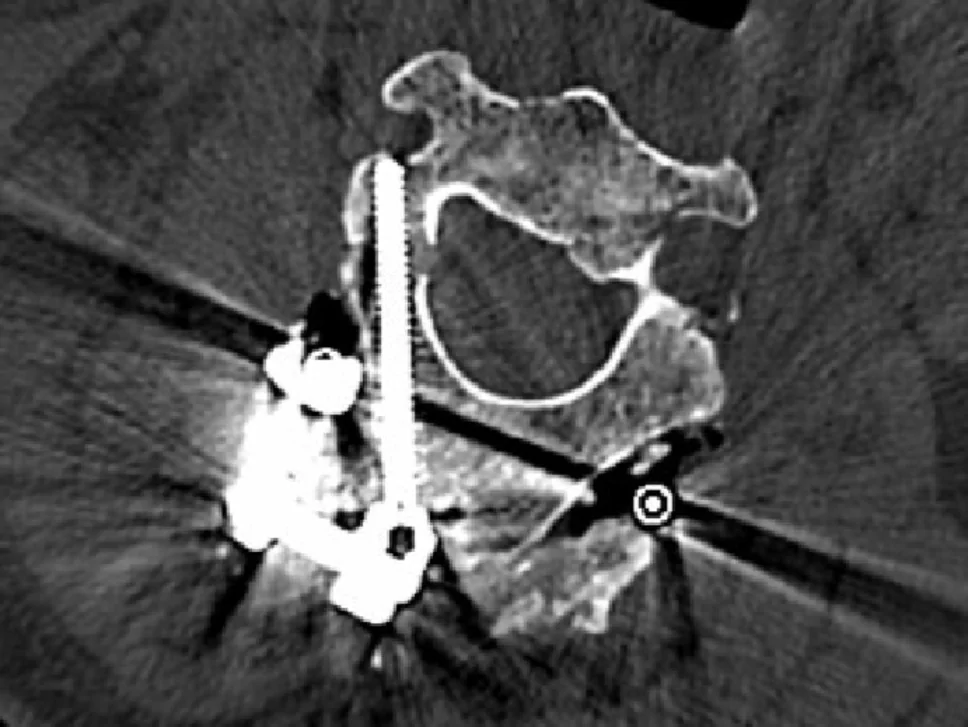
Axial CT pedicle screw C2-rt. (same patient as in figures 3–4 and 6–9). *C*,* cervical spine*
